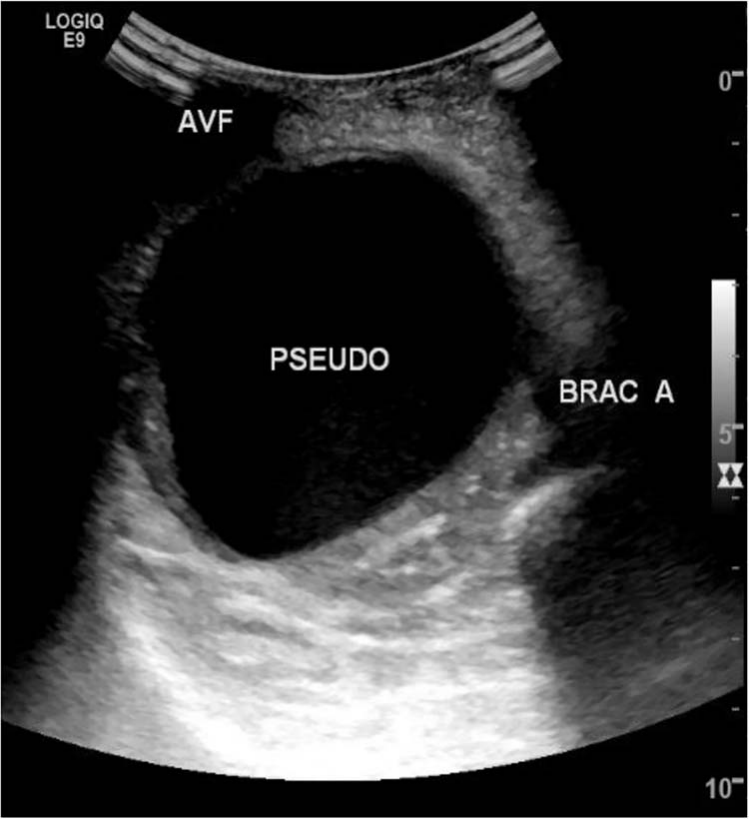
Duplex ultrasound of brachial artery pseudoaneurysm.
